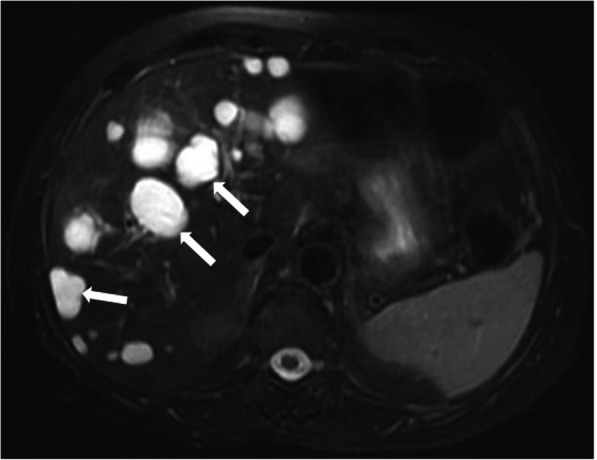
A 60-year-old female patient with known long-standing PLD. Axial plane T2W fat-saturated MR image shows multiple parenchymal cysts in the liver (arrows)
